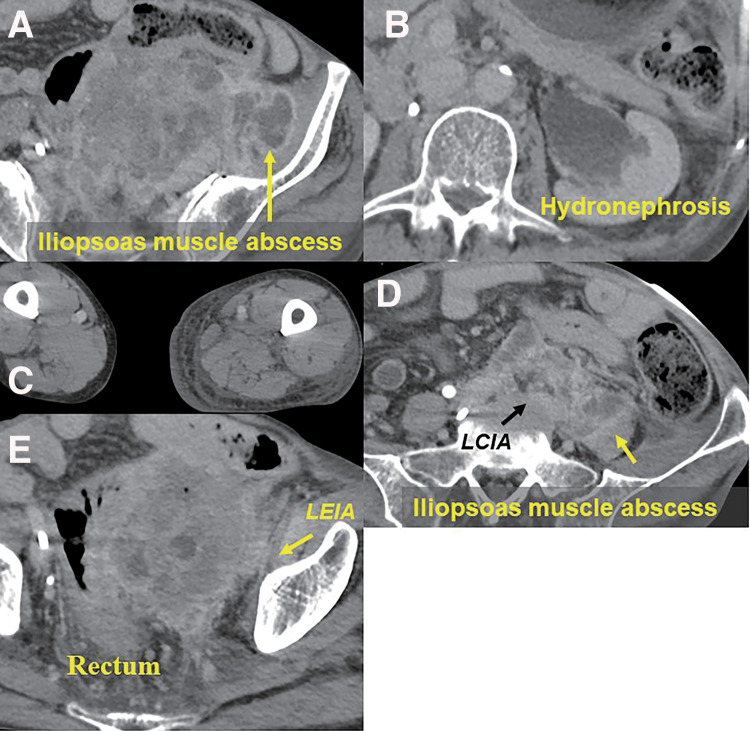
Initial CT revealed an initially unresectable sigmoid colon cancer. The tumor had invaded the iliopsoas muscle and was accompanied by intramuscular abscess formation (A), left hydronephrosis due to left ureteral invasion (B), urinary bladder invasion, and congestive edema of the left lower leg (C) due to invasion of the common to the external iliac artery/vein (D and E). CT; computed tomography, LEIA; left external iliac artery, LCIA; left common iliac artery
